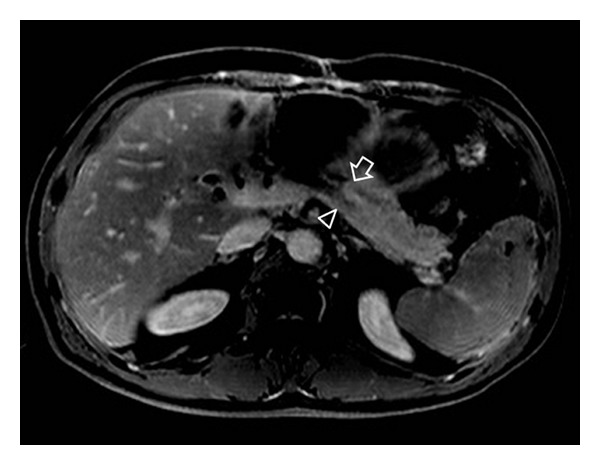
Postoperative followup dynamic magnetic resonance T1-weighted imaging of the remnant pancreas depicting the main as well as the secondary pancreatic duct (arrow and arrowhead resp.) draining the remaining dorsal pancreas at the level of the double pancreaticojejunal anastomosis.
